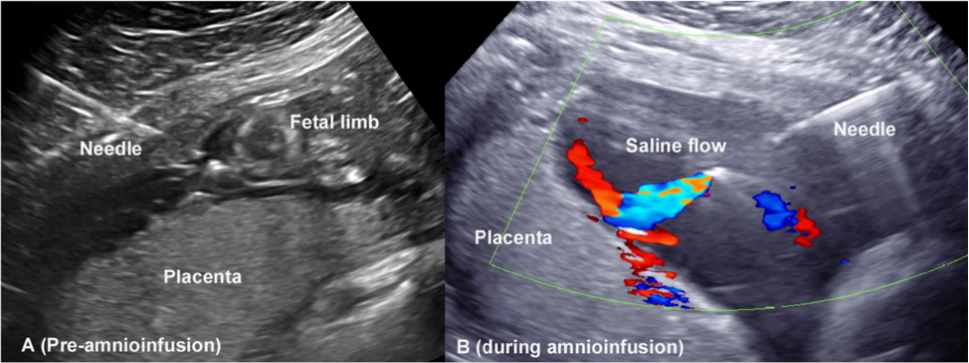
a pre-amnioinfusion insertion of an echo-tip 22 gauge needle into the amniotic cavity (*pocket of loops of cord adjacent to fetal limb*), and b) Color Doppler imaging demonstrating amnioinfusion using sterile saline
